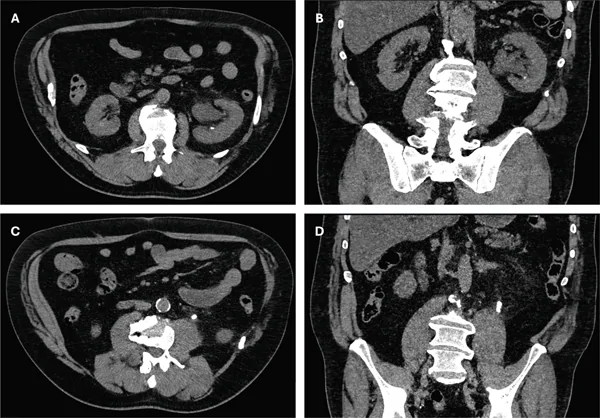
Noncontrast CT urinary tract identifying small left renal stones measuring between 2.6 and 5.0 mm and left‐sided proximal ureteric stones measuring up to 9.5 mm. Average stone density calculated as 650 Hounsfield Units. No right‐sided renal or ureteric stones identified. (A) Axial views of both kidneys with left renal calculi and evident hydronephrosis. (B) Coronal views of both kidneys, showing left renal calculus and hydronephrosis. (C) Axial view of proximal ureters, with left ureteric calculus present. (D) Coronal view identifying multiple proximal left ureteric calculi. *CT protocol details: noncontrast; helical scan mode; slice thickness 0.625 mm; 57 mAs; 120 kVp.*
